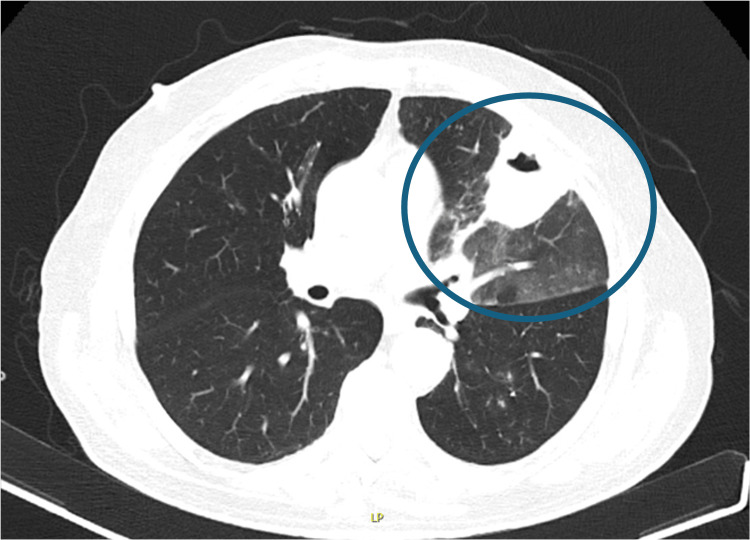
Axial chest CT demonstrating left upper-lobe consolidation with a well-defined cavitary lesion and surrounding parenchymal inflammation (blue circle)
